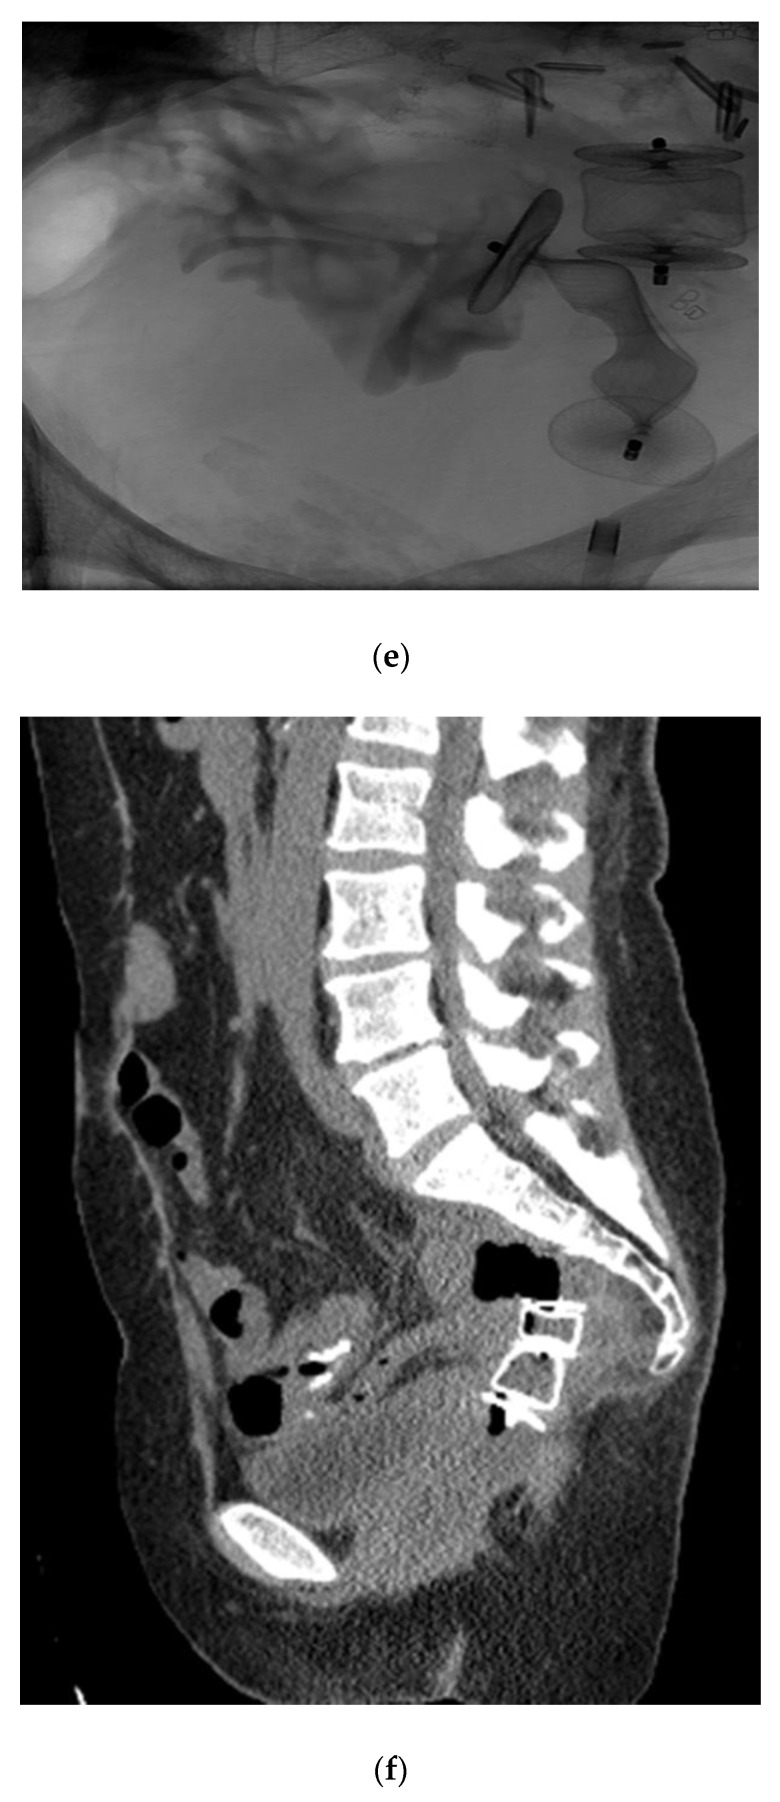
(a) Sagittal T2 MR shows a large fistula (arrow) between a recurrent mucinous rectal tumor (T) and the vagina (V). The rectum is surgically absent; (b) a guidewire has been passed through the vagina into the fistula with the tumor and colon. Contrast is seen opacifying the cecum and ascending colon; (c) the first Amplatzer device is being deployed; (d) the second Amplatzer device is being deployed; (e) after the Amplatzer device is deployed, contrast is retained in the small bowel and no longer communicates with the vagina; (f) a sagittal CT image shows the two adjacent Amplatzer plugs within the fistula tract.
